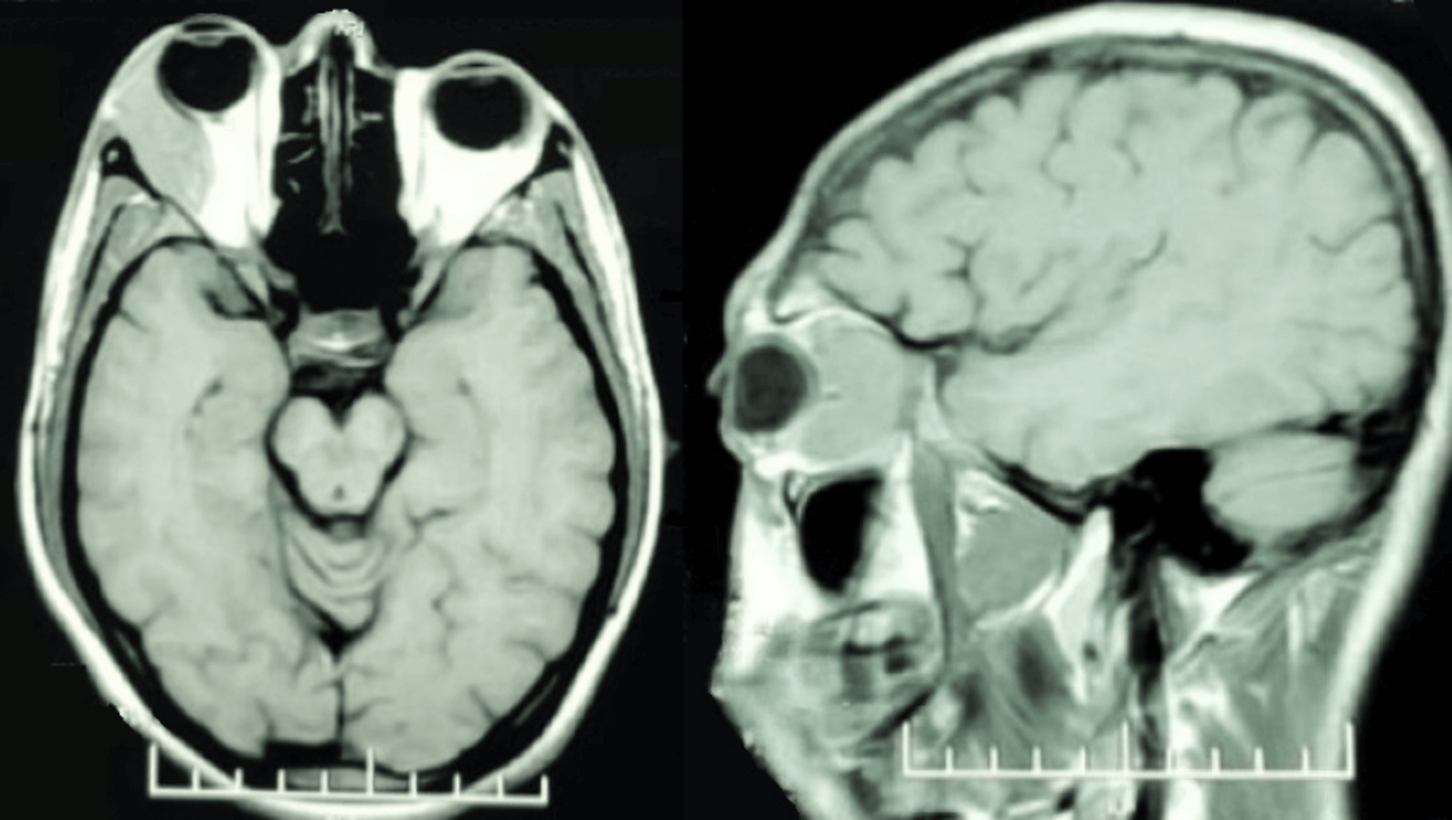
T1-weighted MRI showing the lesion behind the right eye
